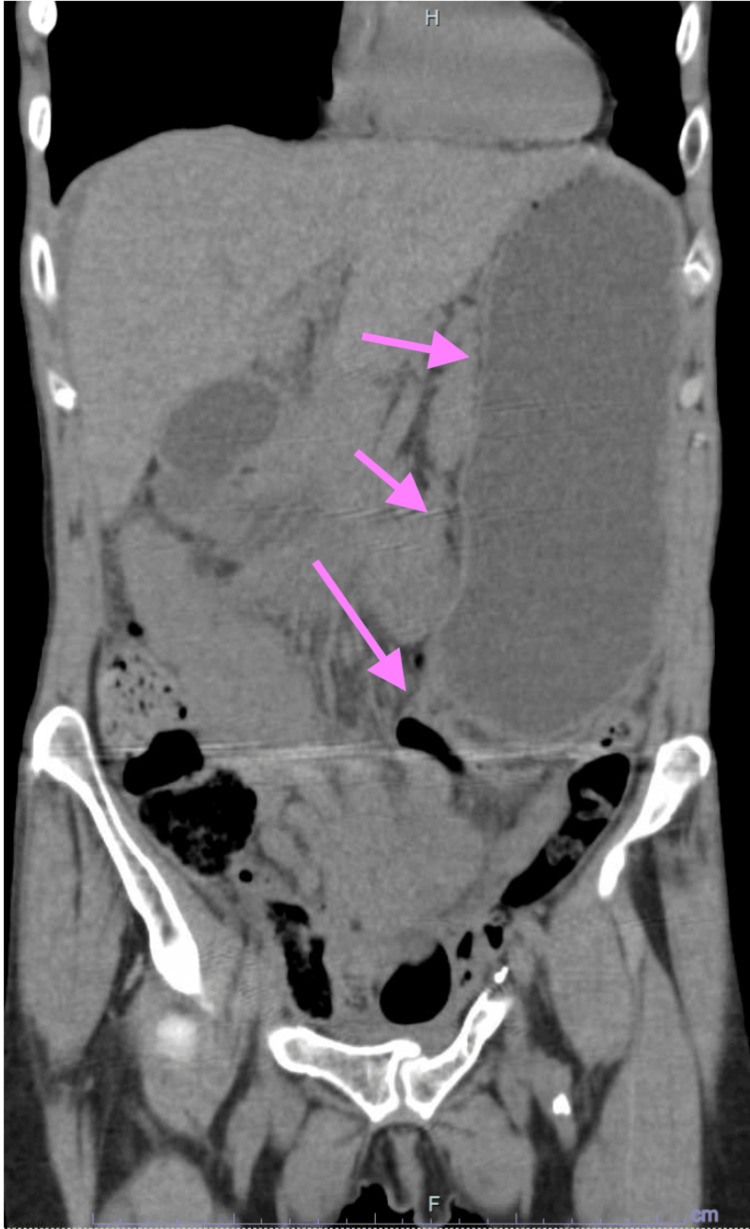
CT of the abdomen/pelvis without contrast showing significant distention of the stomach and proximal-mid duodenum. There is a changing caliber as the duodenum crosses the inferior vena cava. Evaluation for masses limited without intravenous contrast.
